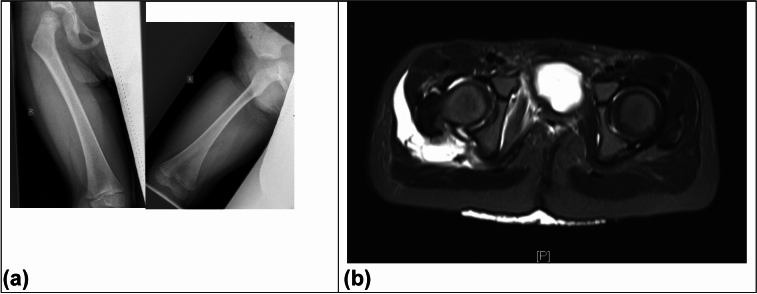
Patient 1: a Immediately after trauma biplanar X-ray in: Suspected epiphysiolysis; b > 24 h after trauma MRI after reduction
